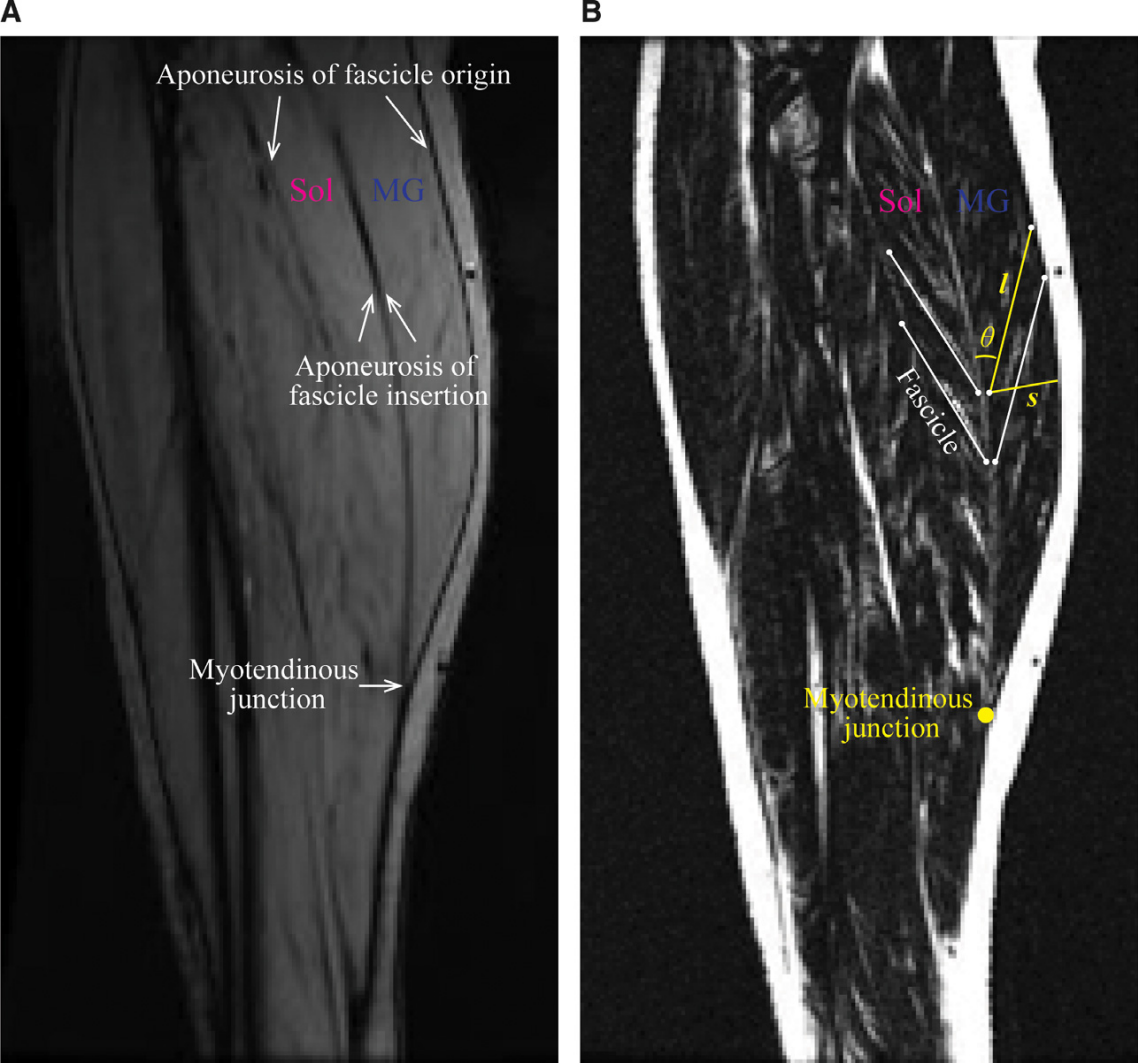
Representative magnitude image from a velocity-encoded, phase-contrast (PC) sequence (A), and water-saturated spin-lattice relaxation time (T1)-weighted image (B). The water-saturated T1-weighted image allowed one to identify the origin and insertion of the muscle fascicles, as shown by white dots. Those dots and the connecting lines were registered onto the PC magnitude images for displacement tracking of the fascicle and the subsequent calculation of fascicle length (*l*), pennation angle (*θ*), and thickness (*s*). The fascicle length was measured as the distance from the two ROIs identifying the fascicle origin and insertion points. The pennation angle was calculated as the angle between the line defined by the fascicle and the tangent at the aponeurosis at the point of fascicle insertion. The thickness was defined as the length of the normal from the point of fascicle insertion to the opposite aponeurosis.
